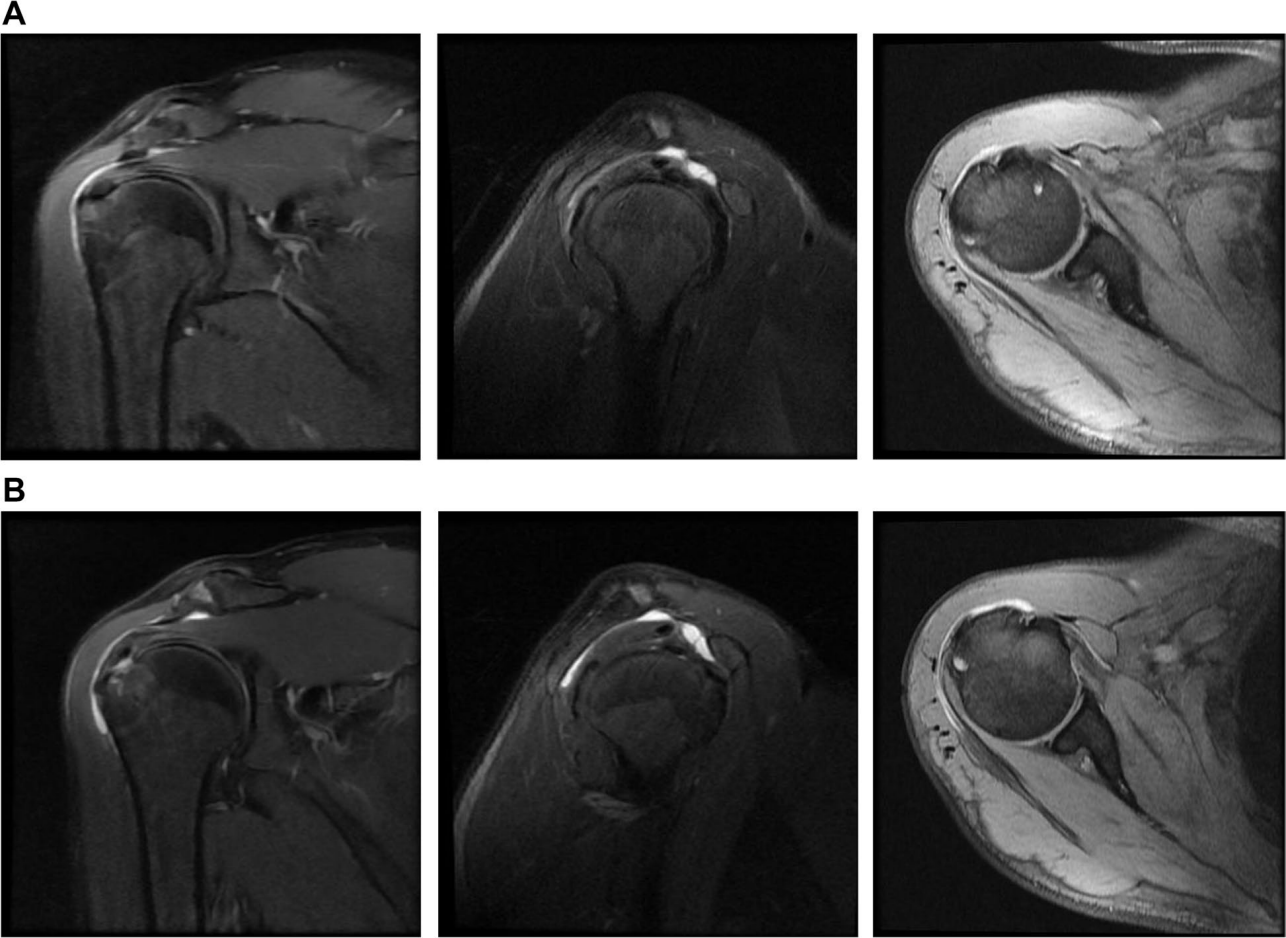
MRI images of a 47-year-old male patient. A Images of bursitis with intact rotator cuff before vaccination. B Post-vaccination images show increased bursitis and partial rotator cuff tear
